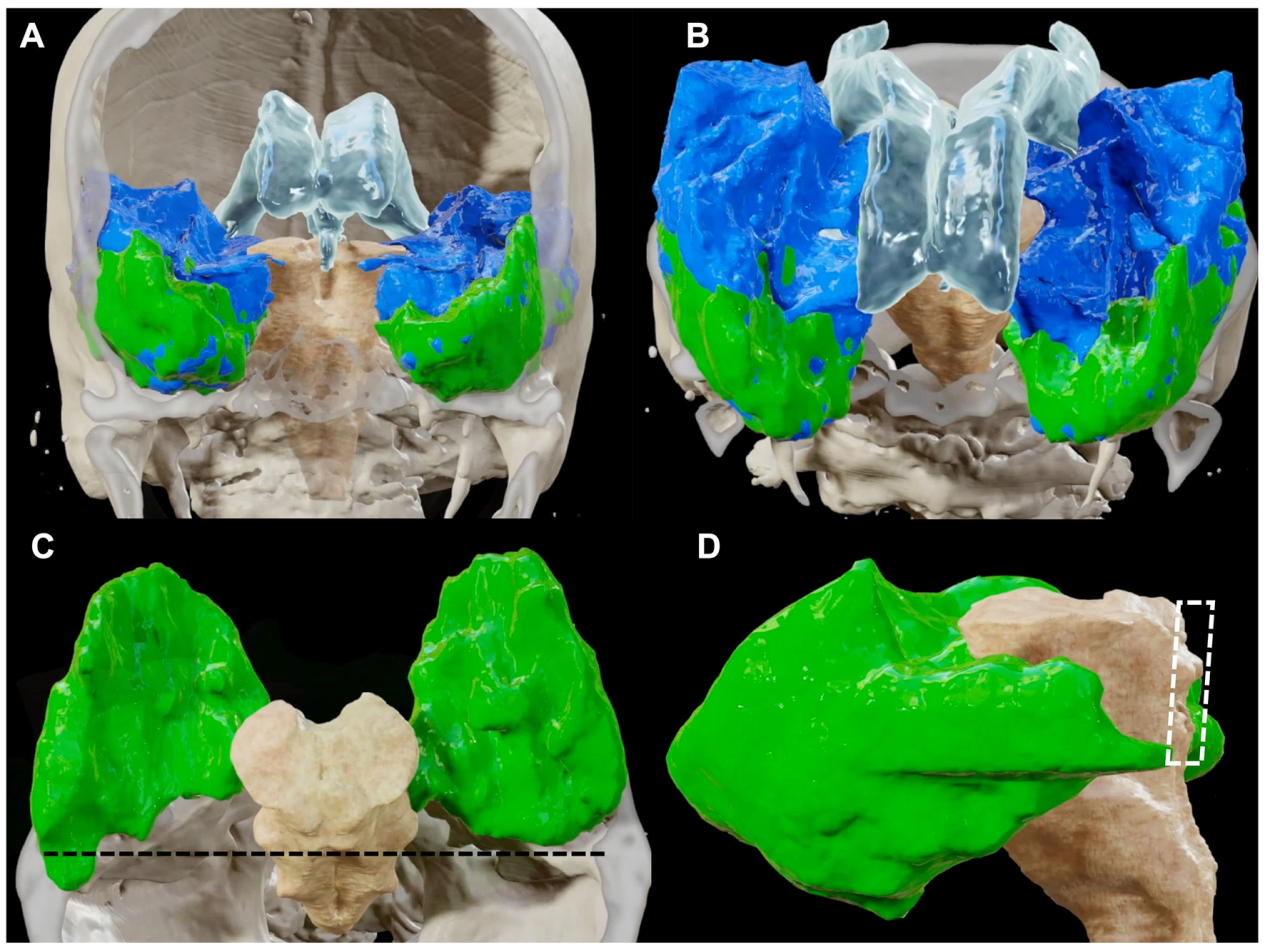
BrainLab^®^ reconstructions retrieved from post-dissection MRI quantifying the amount of temporal lobe removed (in green) in relation with the intact temporal lobe (in blue), seen from an anterior (A) and superior (B) perspective. In (C) and (D), only the temporal lobe removed was left to highlight the relationships of the posterior limits of the resection with the surrounding brain structures. The posterior limit of the resection is represented by a plane tangential to the lamina quadrigemina [dark dotted line in (C) and white dotted area in (D)] as it is demonstrated in a superior (C) and lateral (D) view. The qualitative analysis of our resection shows that while the middle and inferior temporal gyruses together with the basal and medial portion of the temporal lobe represented the largest amount of the resection, the posterior two-thirds of the superior temporal gyrus were left intact.
